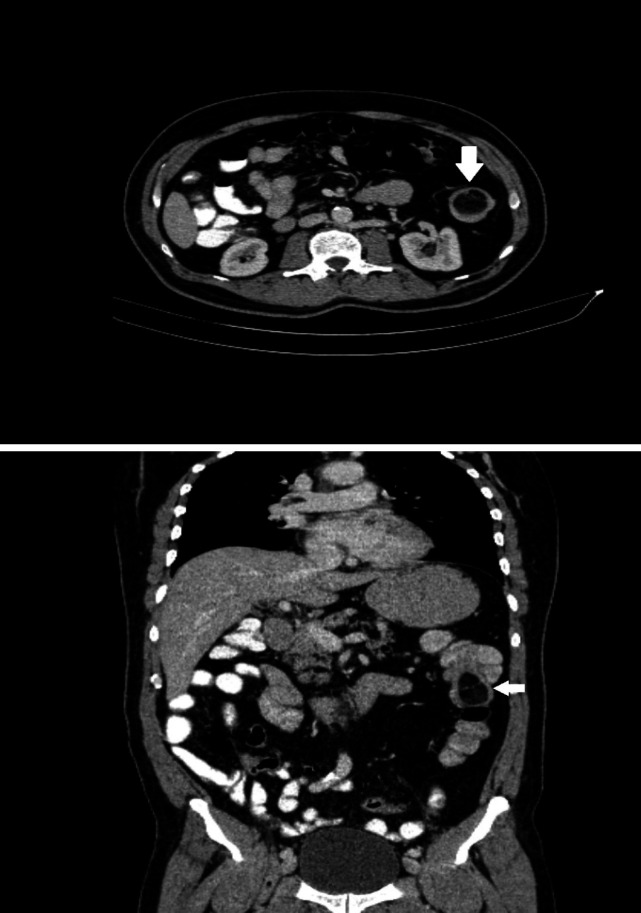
A mass on the descending colon (white arrow) with a density of approximately -100 Hounsfield units on computed tomography (CT)
